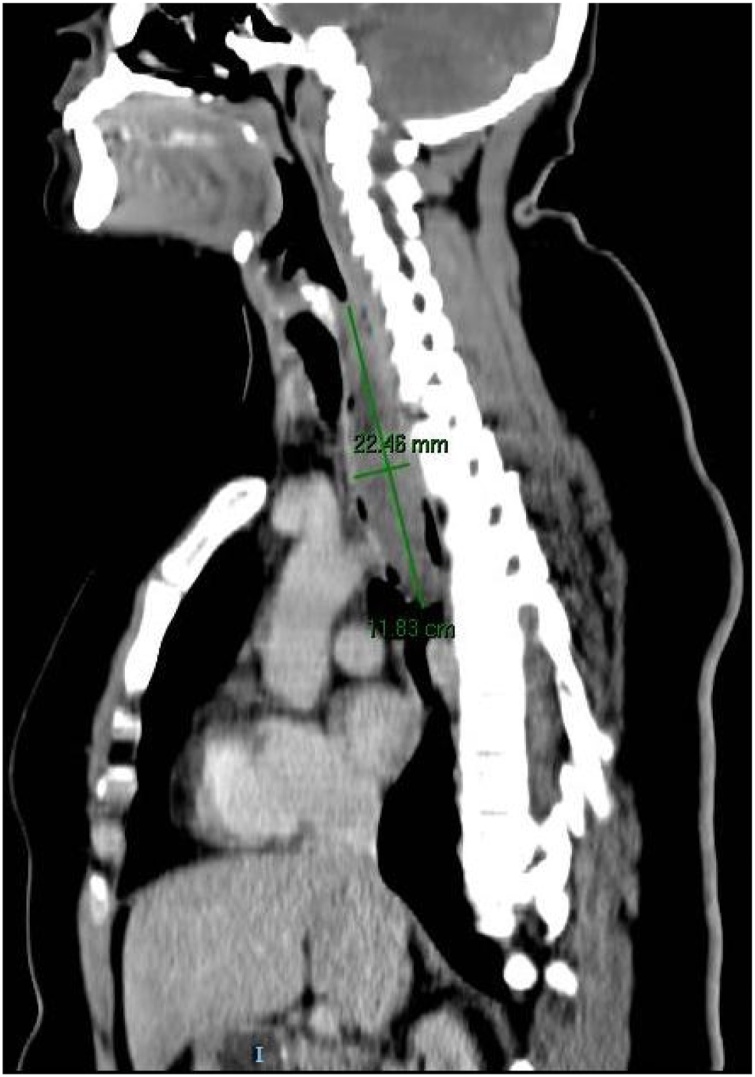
Computed tomography scan image showing a multilobulated esophageal tumor.
